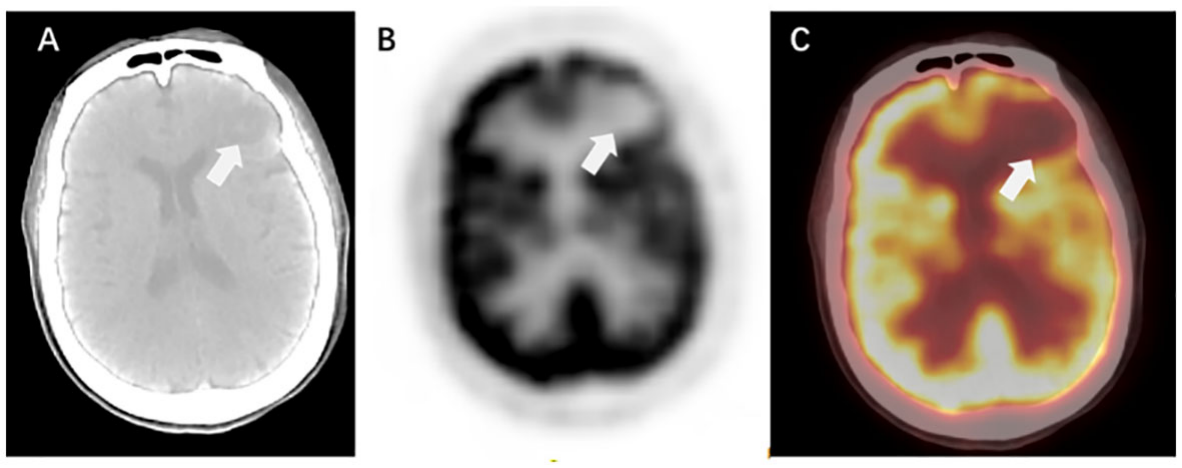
PET/CT manifestations of BMs. [(A) CT without contrast; (B) PET; (C) PET/CT].
